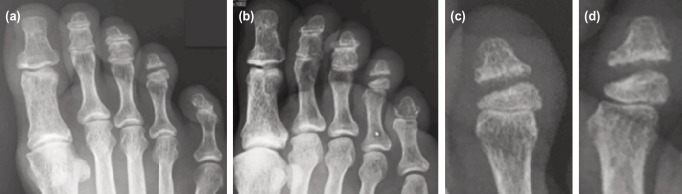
Pre-operative radiographs of fracture non-union of right fourth toe distal phalanx. (a) Dorso-plantar foot view, (b) Oblique foot view, (c) Magnified dorso-plantar fourth toe view, (d) Magnified oblique fourth toe view.
